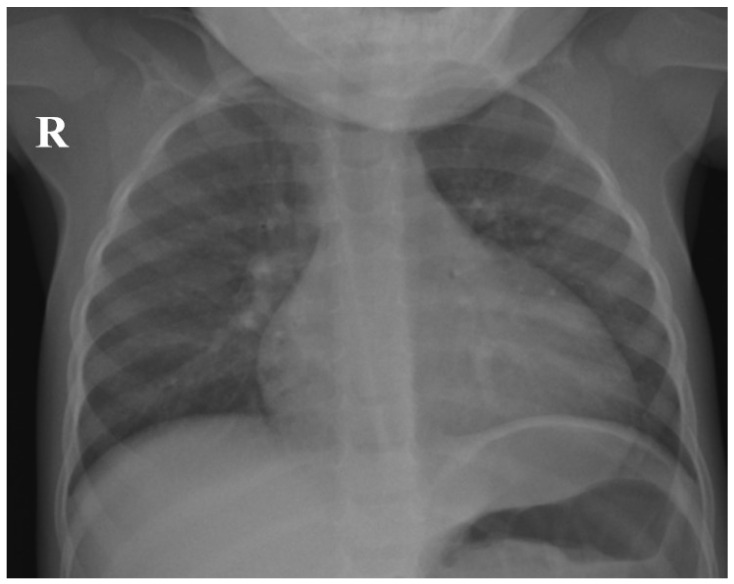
Chest X-ray of the patient demonstrating bilateral peribronchial interstitial shadowing, consistent with viral pneumonitis (R—right side of the patient).
